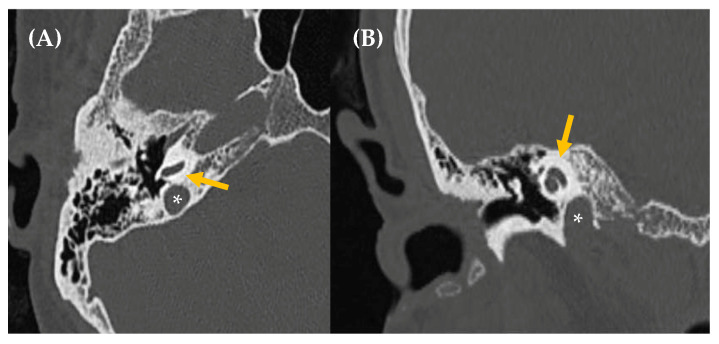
CT imaging (image (A), axial section/image (B), coronal section) reveals asymmetry in size between both jugular bulbs, more prominent on the right side, which reaches a slightly higher position than the contralateral one, up to the height of the basal turn of the cochlea, without reaching the height of the IAC. The jugular wall is continuous, with no evidence of dehiscence (asterisk: jugular bulb/yellow arrow: cochlea).
